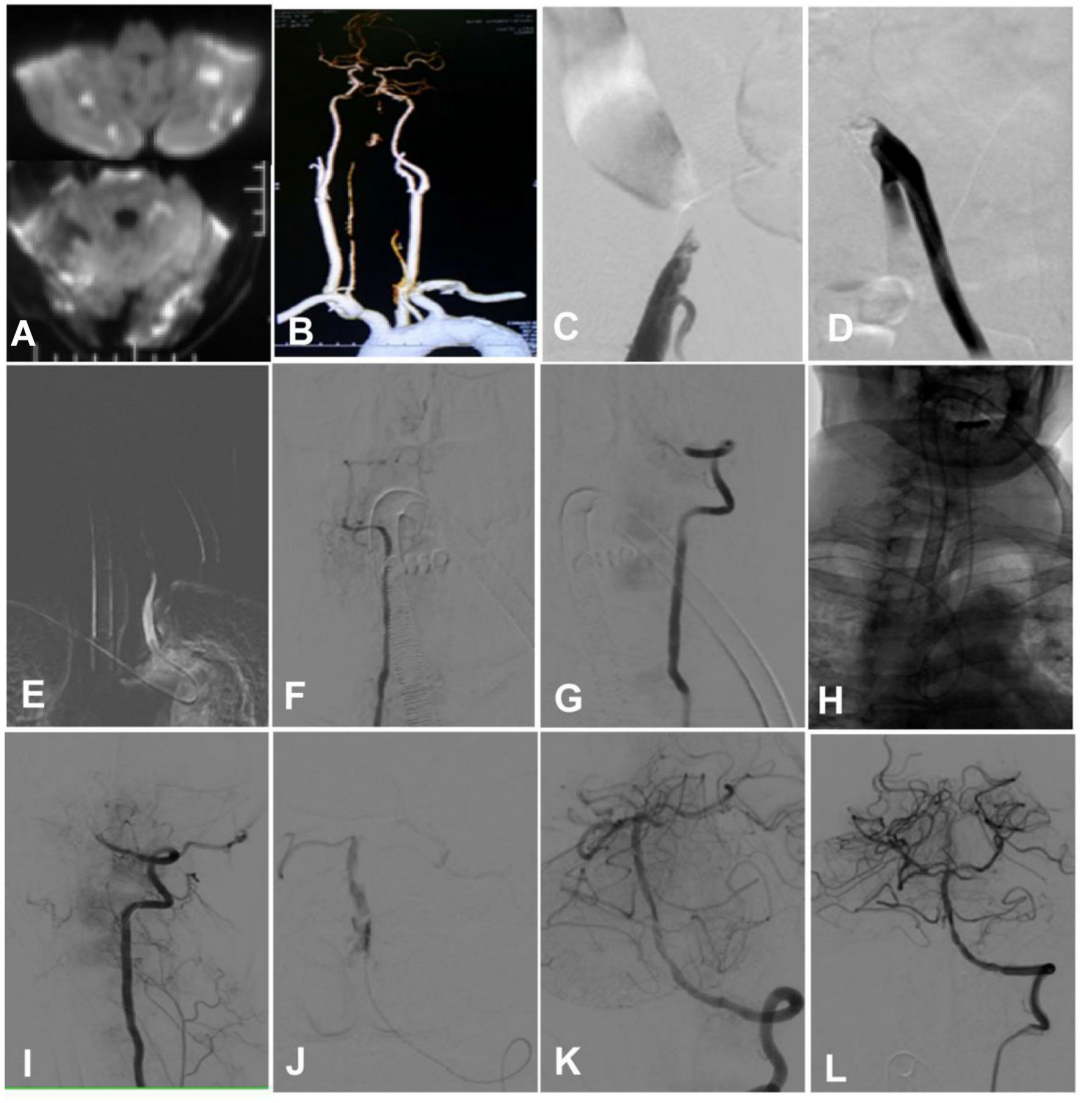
A man in his early 50s with hypertension for 20 years and diabetes mellitus for 20 years was admitted because of tetraplegia for 12 h and dizziness and diplopia for 2 days. (A) A magnetic resonance imaging scan showed multiple fresh infarcts in the bilateral cerebellum, the occipital lobe, and the brain stem. (B) Computed tomographic angiography revealed bilateral vertebral artery intracranial segment occlusion, with no display of the basilar artery. (C) Angiography through the right femoral artery demonstrated occlusion of the right iliac artery. (D) Angiography via the left femoral artery indicated occlusion of the left iliac artery. (E–G) Cerebral angiography through the right radial artery angiography showed occlusion of the bilateral vertebral artery intracranial segment. (H) After accessing through the right radial artery, a guiding catheter was sent to superselect the left vertebral artery after looping in the aorta. (I) The tip of the guiding catheter was navigated to the V3 segment of the left vertebral artery. (J) With the help of a Synchro-14 micro-guide wire (Stryker, Salt Lake City, Utah, USA), an Echelon-10 microcatheter (Medtronic, Heerlen, Netherlands, USA) was navigated through the occluded segment for angiography, and it showed good patency of the distal arteries. (K, L) A Gateway 2.0 × 15 mm balloon catheter (Stryker Fremont, CA, USA) was used to dilate the occluded segment before deploying an Enterprise 4.5 × 22 mm stent (Cordis, Chaska, MN, USA) at the segment. Angiography revealed good blood flow in the vertebral and basilar arteries.
